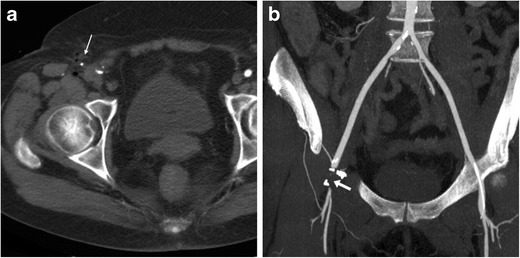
Segmental occlusion of the punctured right CFA in a 65-year-old woman suffering from acute lower limb ischaemia following transarterial mitral valve replacement. Despite inconclusive findings on precontrast CT images (thin arrow in a, note similarity with Fig.1), CT angiography (B) showed non-opacification of a 2-cm segment of the CFA (arrow). Surgical arteriotomy and endoarterectomy was immediately performed
